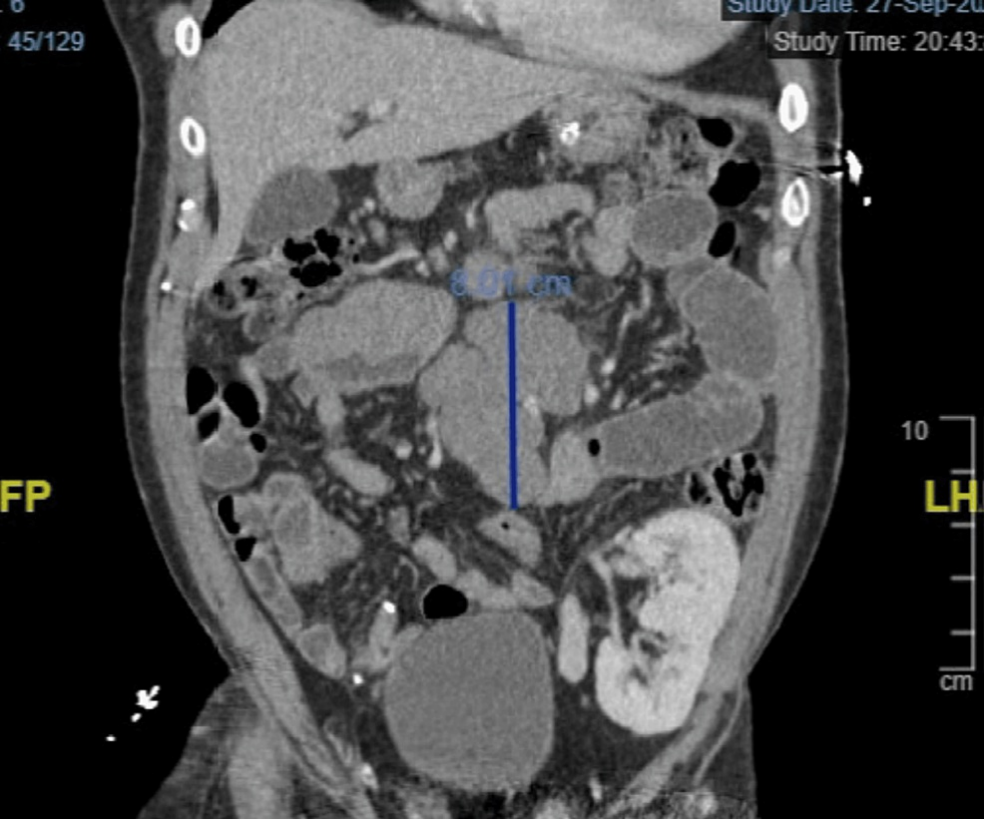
CT of the abdomen and pelvis with iodinated contrast demonstrating a 7 x 5 cm mass in the distal jejunum (highlighted with a vertical line).
